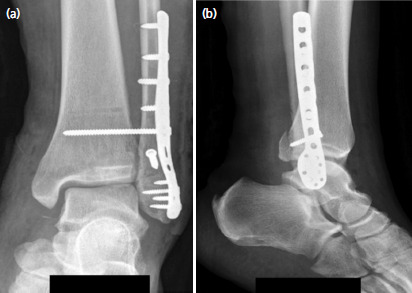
Patient 2 – Post-operative (a) antero-posterior and (b) lateral radiographs of the ankle at six weeks.
